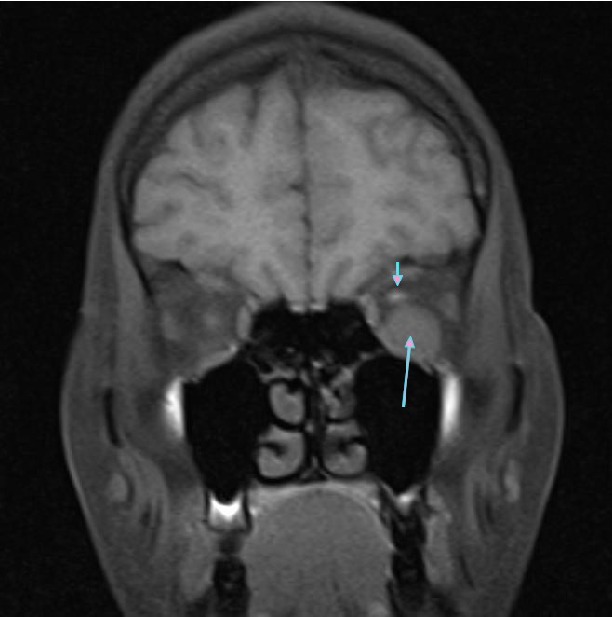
Coronal T1 weighted MR image showing hypointense haematoma (long arrow) and superomedially displaced left optic nerve (short arrow).
